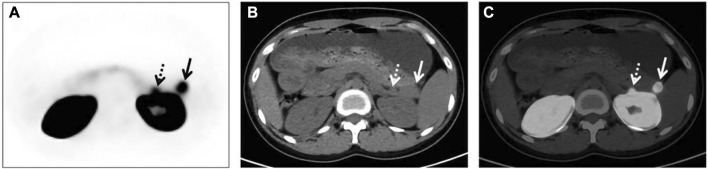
GLP-1R molecular imaging of double-primary insulinomas. Two insulinoma lesions in the tail of the pancreas, only one of which was detected in conventional imaging.68 Ga-DOTA-exendin-4 molecular imaging successfully detected both lesions and guided surgical resection. In the axial images [(A) PET, (B) CT, (C) fusion) in addition to the significantly higher uptake of the lesion in the tail of the pancreas, we see that GLP-1R imaging successfully detected the other lesion adjacent to the kidney, which was not detected in the MRI image due to the obscuration of the kidney. Thus, GLP-1R molecular imaging offers better advantages over conventional imaging in the preoperative precise localization of insulinomas.
